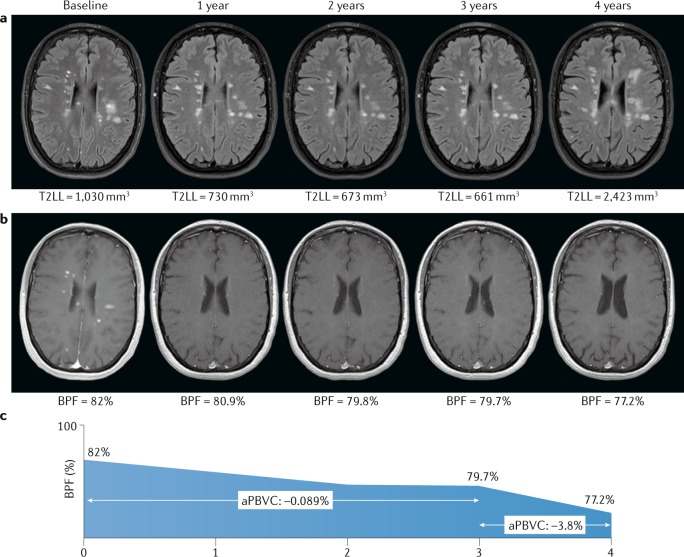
Lesion load and brain atrophy in relapsing–remitting multiple sclerosis. a | Transverse T2-weighted fluid attenuation inversion recovery images from a patient with highly active relapsing–remitting multiple sclerosis (MS) who started a disease-modifying therapy at baseline. The T2 lesion load (T2LL) is stable during the first 3 years of treatment while the patient remained clinically stable (no relapses and no disability worsening), but markedly increases at the fourth year after treatment discontinuation associated with clinical activity (the rebound effect). b | Contrast-enhanced T1-weighted images from the same patient showing the change in brain parenchymal fraction (BPF) over time. The decrease in global brain volume in the first 3 years is mild (annualized percentage of brain volume change (aPBVC) −0.089%), but the volume loss at the fourth year is severe (aPBVC −3.8%), matching the change in T2LL and clinical evolution. The severe loss observed in year 4 is well beyond the −0.4% suggested as a pathological cut-off for brain volume loss in MS^24^. c | Graphical representation of the changes in BPF over time, emphasizing the dramatic loss of volume in year 4.
